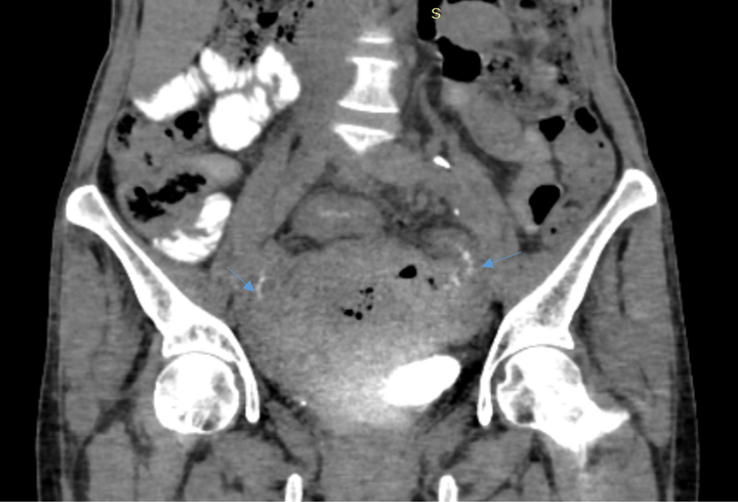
Coronal section of computed tomography (CT) of abdomen and pelvis with rectal contrast showing a thin rim of contrast passing from the rectum to jejunal loops on bilateral sides via the fistulas (thin blue arrows).
